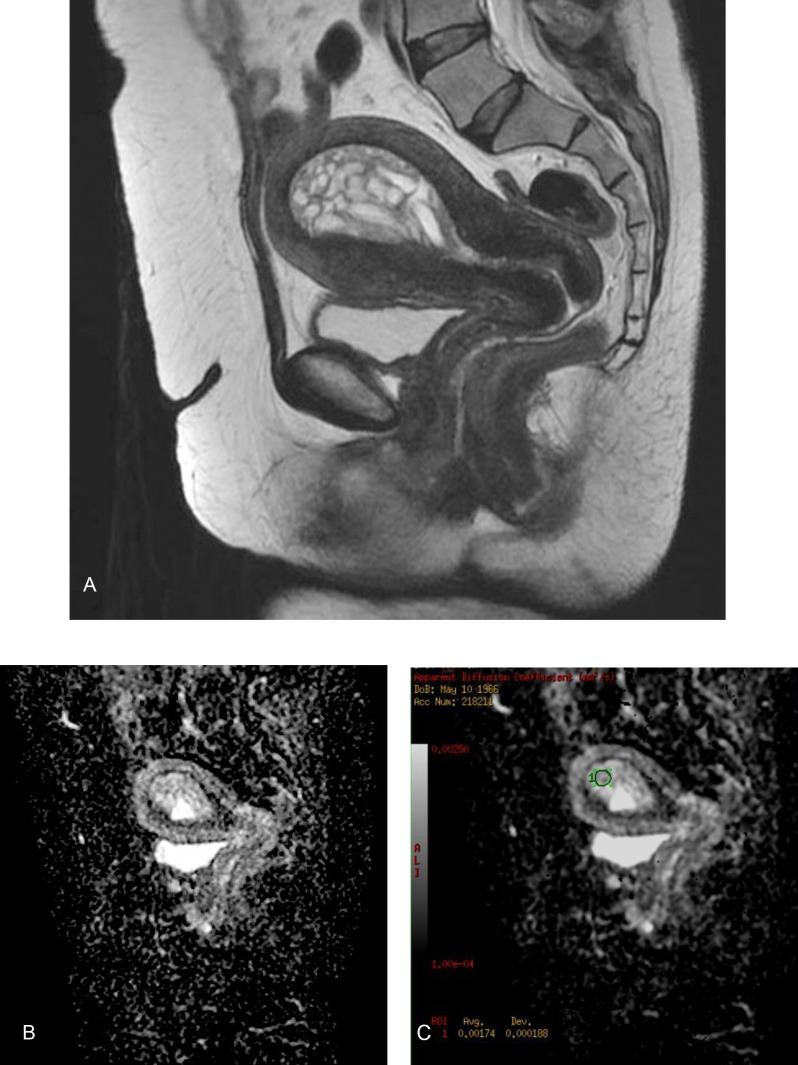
A 47-year-old woman with benign endometrial hyperplasia. Sagittal T2-weighted fast spin-echo image (a) shows diffuse endometrial thickening, and hyperintense cysts are seen in the endometrial cavity. Sagittal ADC map image constructed from diffusion-weighted image (b = 1000s/mm^2^) (b). On the ADC map, the endometrial cavity shows a heterogeneously high signal intensity, and the ADC value is 1.74x10^–3^ (c).
